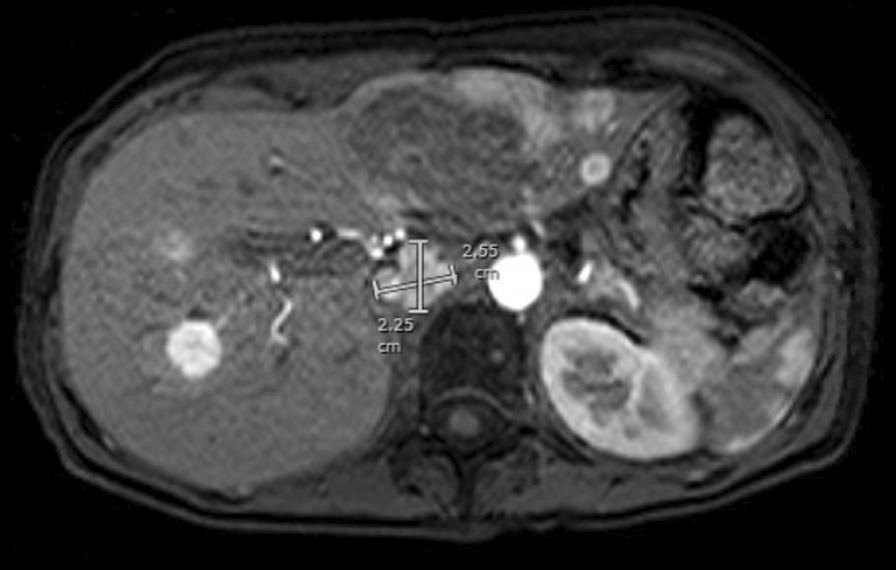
Magnetic resonance imaging of the abdomen with and without contrast. Highlighted is the largest retroperitoneal mass (2.65 × 2.25 cm)
